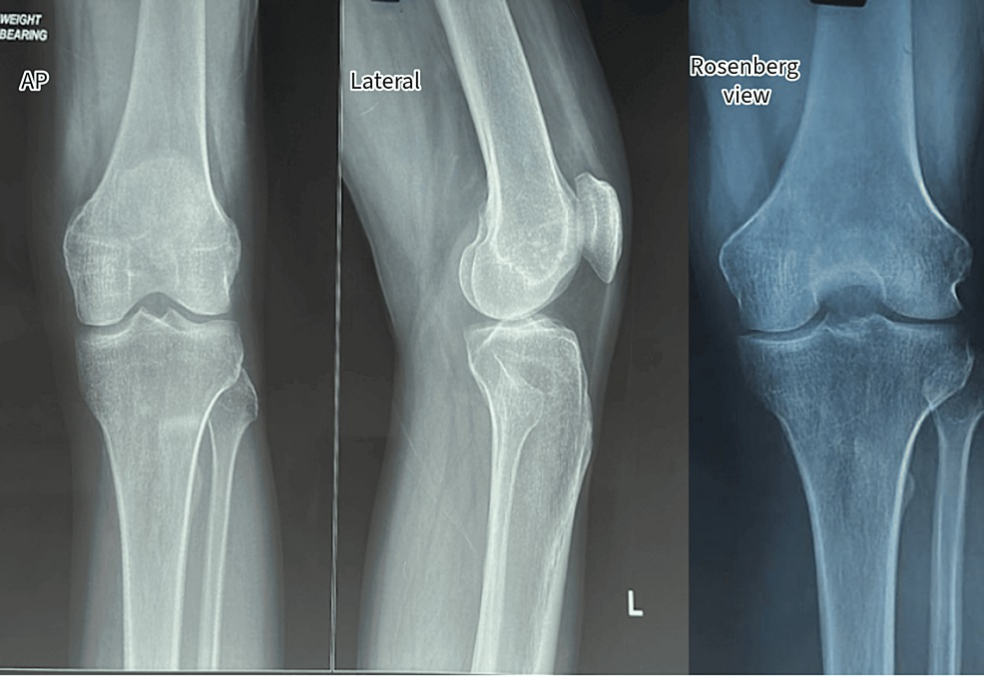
Preoperative plain radiograph
